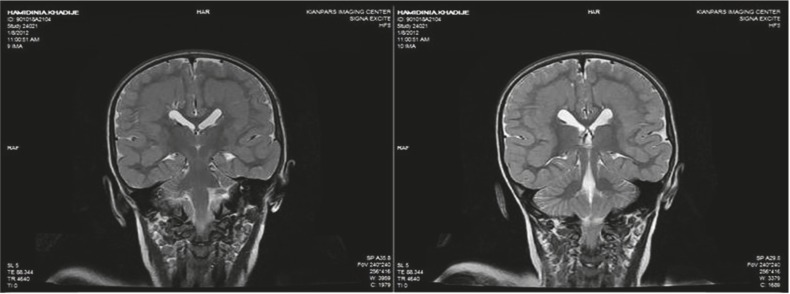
Coronal T2-weighted brain MRI, shows polymicrogyria, complete band heterotopia, and corpus callosal dysplasia
